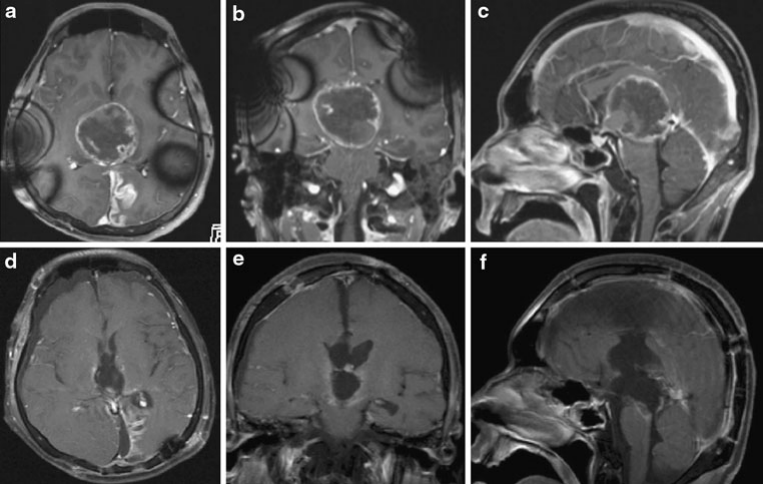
MR images taken during the course. a–c MRI with contrast during radiation showing enlargement of the tumor, indicating growing teratoma syndrome. d–f MRI with contrast after the third surgery showing a residual lesion on the right side of the third ventricle
